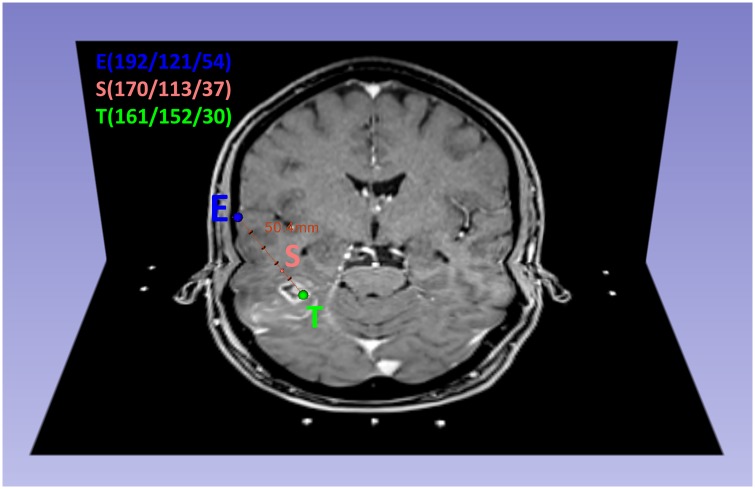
Calculation of the biopsy point S that is located in the enhancing tumor area (CE). Combination of an axial and a coronal slide of intraoperative cT1 MRI in a 60-year-old patient with glioblastoma. Cranial view. The biopsy point S is marked within the NE-area of the tumor. The trajectory (red line, length: 50.4mm) and the biopsy point S (170/113/37) were calculated via vector analysis in MATLAB from the coordinates of the known entry point E (192/121/54) and the target point T (161/152/30). The distance between S and T was 15.0 mm.
